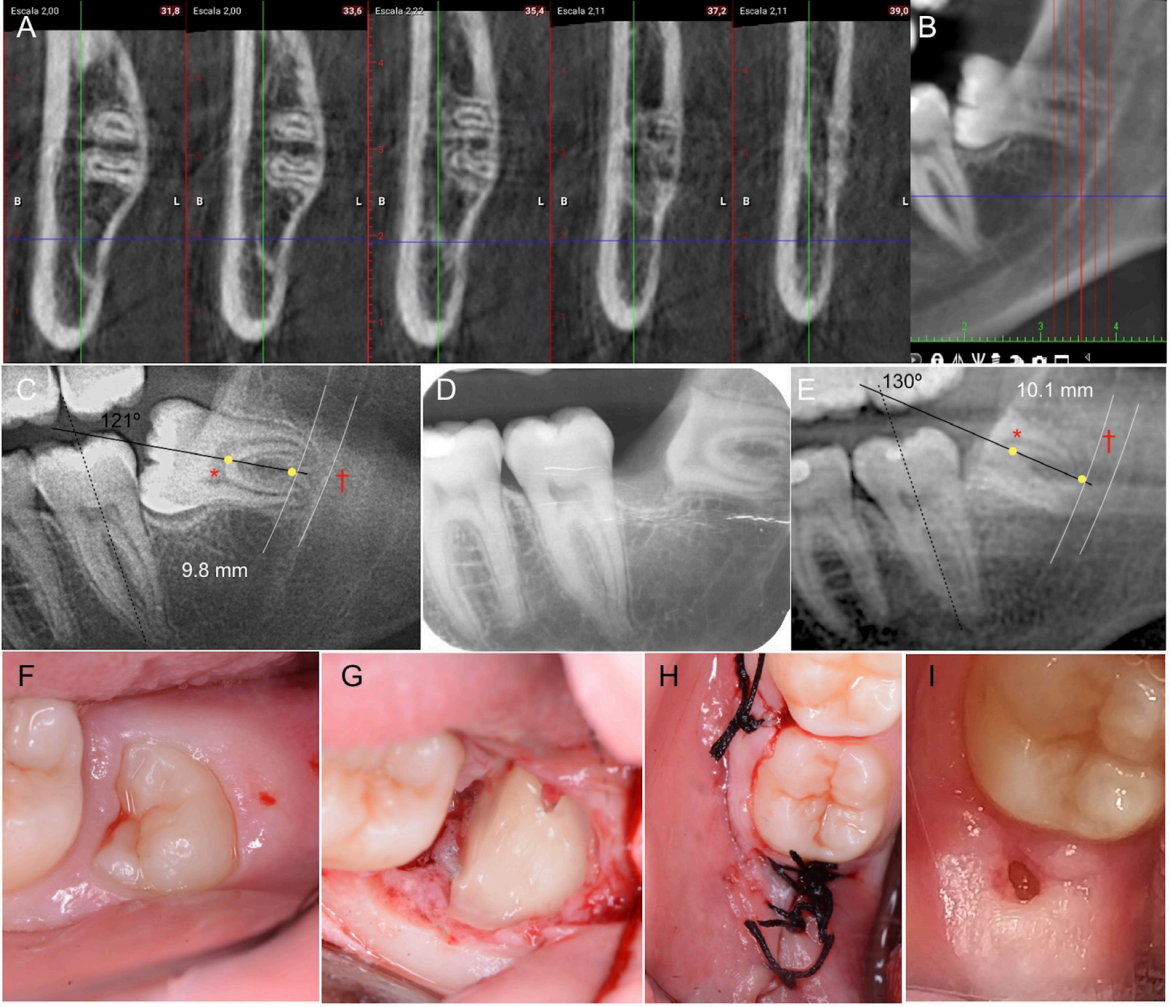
Case no. 1. Initial CBCT. A) Coronal sections separated at 1.80 mm; B) Sagittal section. C) Initial OPG, (D) immediate postoperative periapical, (E) OPG at 12 months follow-up (*Stable reference [most apical part of the pulp chamber] and †point where the axial axis of the LTM contacts the upper part of the IAN canal). F) Initial intraoral clinical image of 3.8.; G) Coronectomy of 3.8. H) Immediate postoperative phase; I) Operculitis diagnosed at 12 months follow-up due to coronal migration of the root portion.
